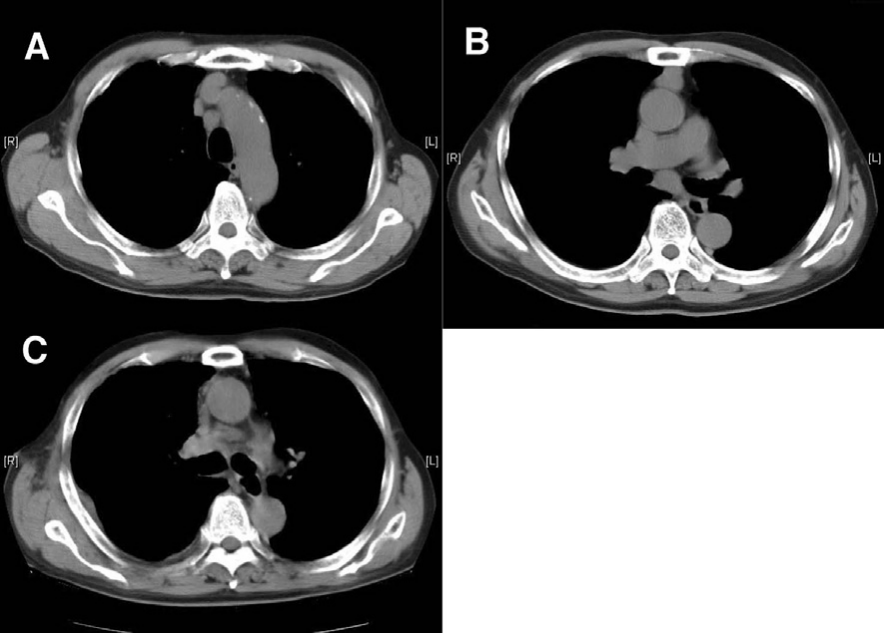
CT scan images of (A) pretracheal lymph node, (B) subcarinal lymph node, and (C) mediastinum after hemorrhage from right pulmonary artery. A small hematoma developed in the pretracheal space. Gauze packing was not done.
